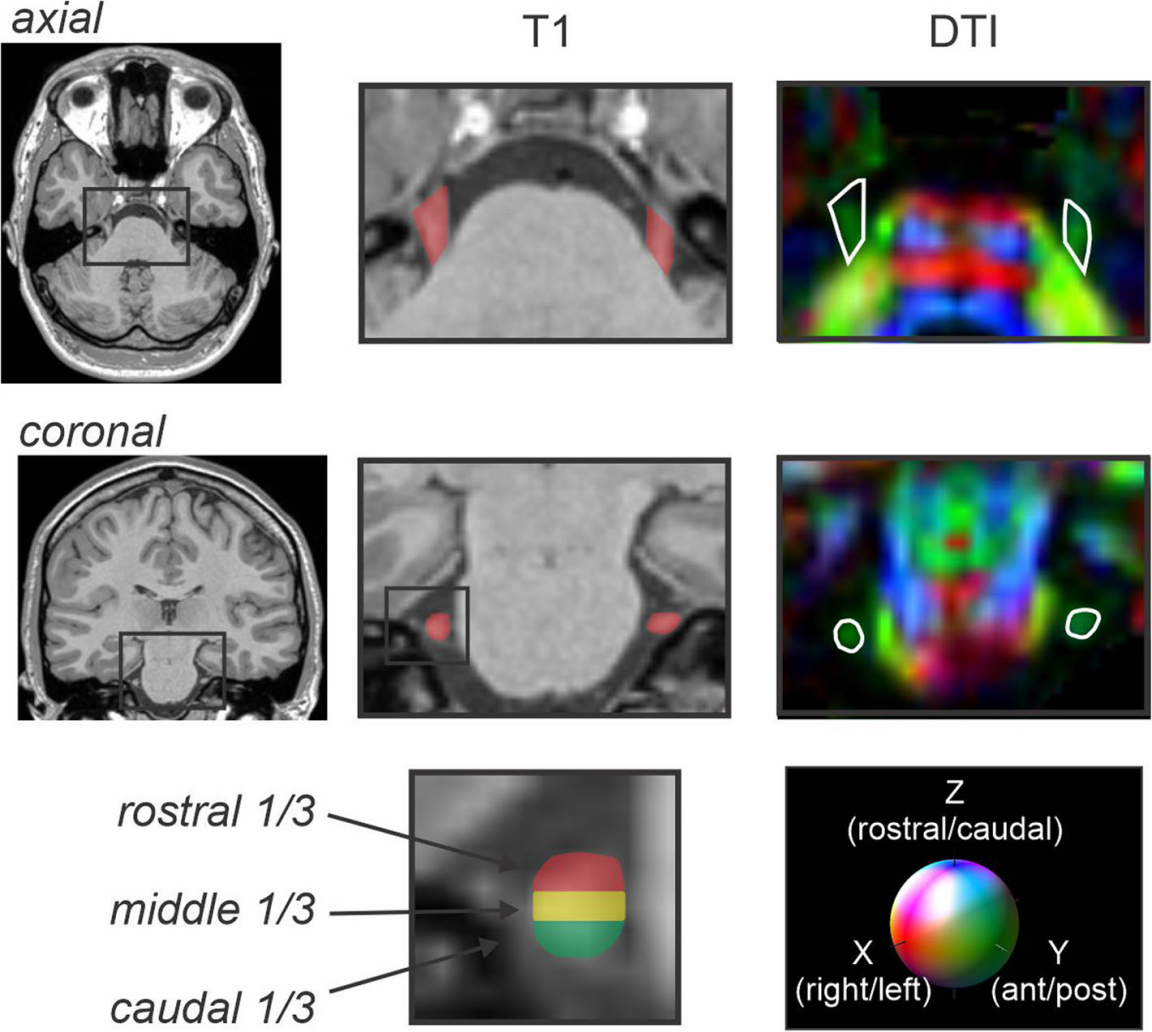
Axial and coronal T1-weighted anatomical images and corresponding diffusion tensor (DTI) images showing the trigeminal nerve root entry zone in a single subject. The DTI image is color-coded for direction of greatest water movement. The outline of the trigeminal nerve region used for total nerve analysis is also shown in red shading on the T1-weighted anatomical image and outlined in white on DTI images. To the right is an example of the regions selected for the rostral (red), middle (yellow) and caudal (green) third of the nerve at the maximal cross-sectional area
